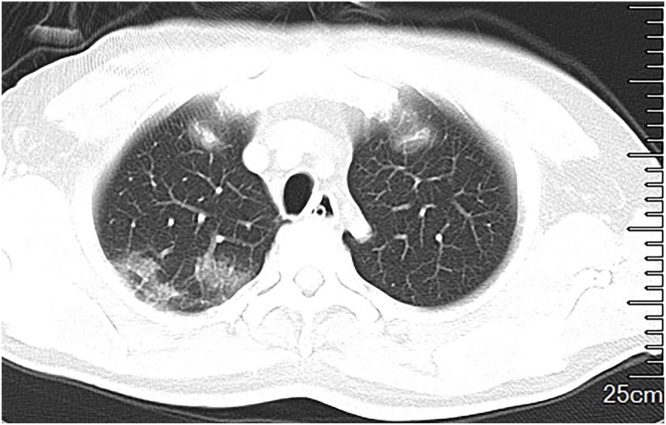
CT of chest shows patchy ground glass opacities in right upper lung, peripheral field.
